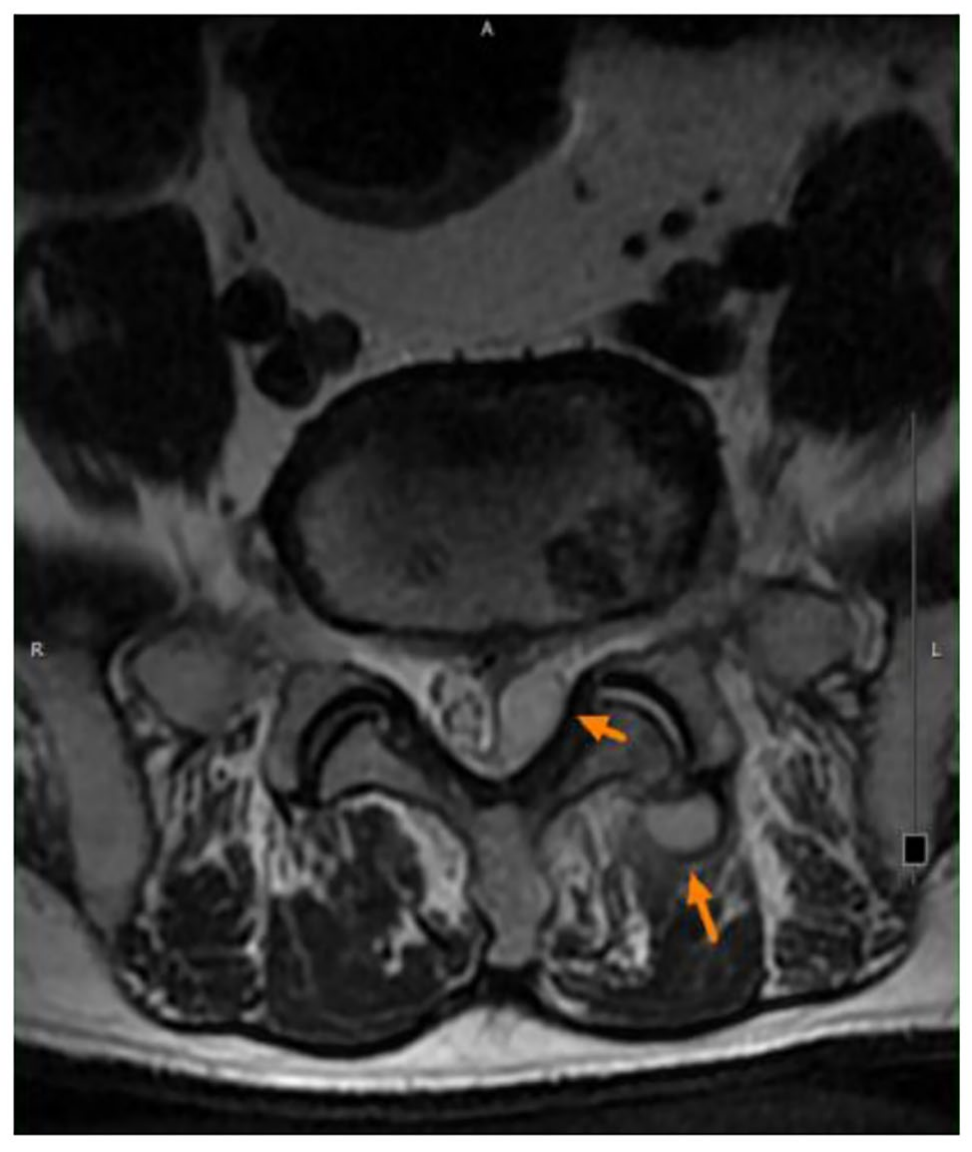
Shows the hyperintense signal of the masses in the T2-weighted image
